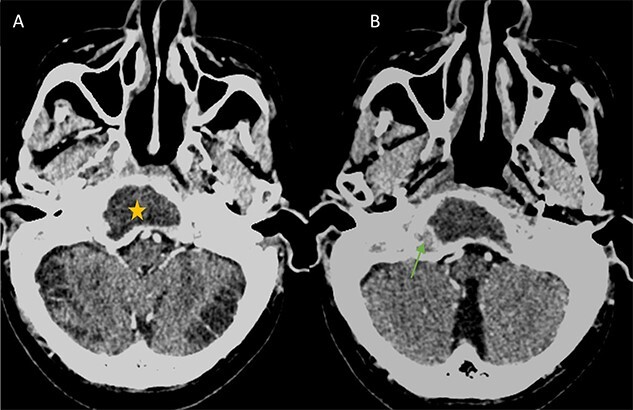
(A) Axial CT in soft-tissue window showing an intra clival fluid collection (star). (B) Axial Post contrast CT showing a peripheral enhancement (arrow).
